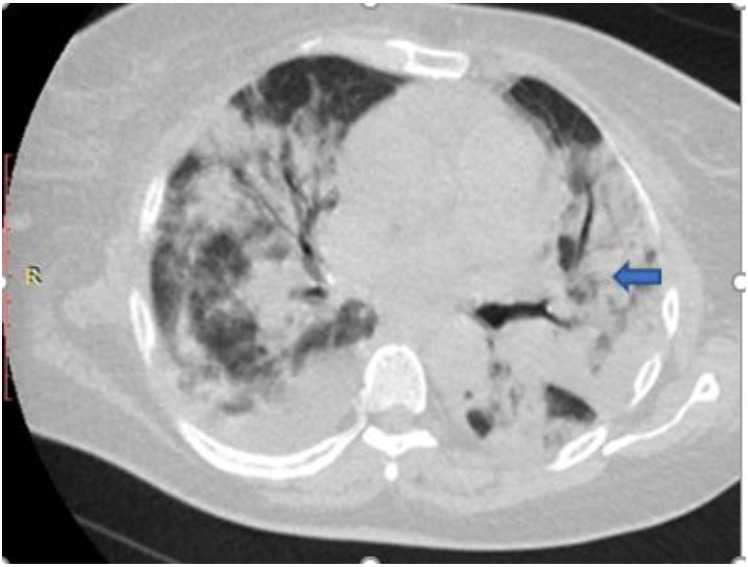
An axial non-contrast enhanced chest CT scan; lung window of a 9-year-old girl with a 10-day history of fever and cough shows dense consolidation (Arrow) in the left lower lung zone, which is not peripherally distributed but instead, more central. Tiny multifocal consolidations and ground glass infiltrates are seen on the right along with a minimal right pleural effusion. Features were categorised as ‘Indeterminate’. RT-PCR, however, confirmed COVID-19.
